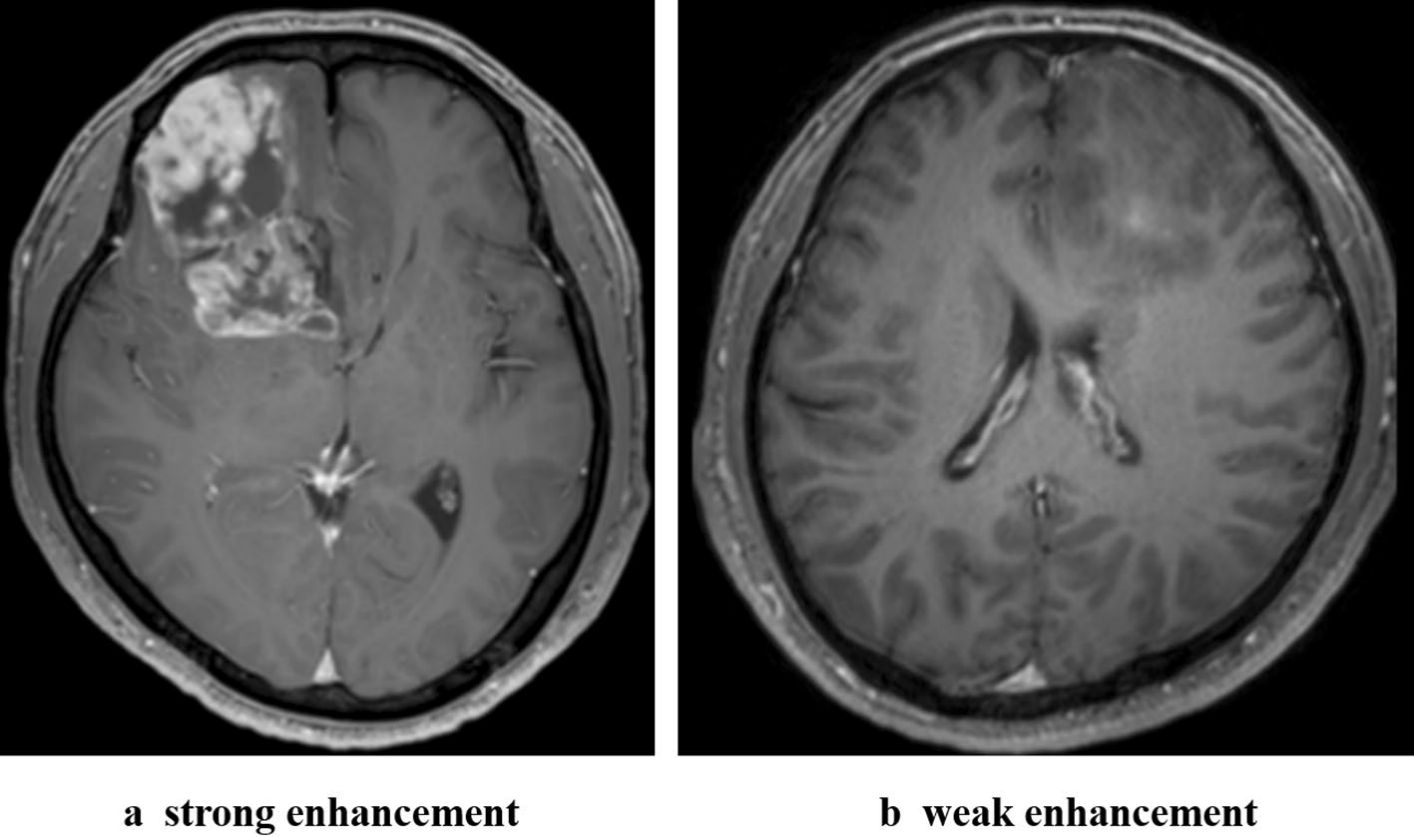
Classification of enhancement pattern according to T1 gadolinium-enhanced MRI.
